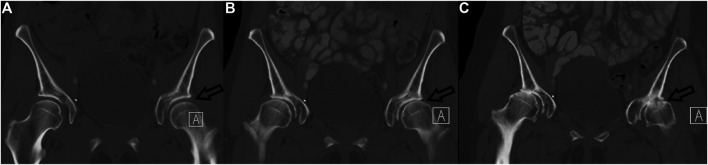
Radiation-related bilateral femoral head necrosis. Three coronal CT images of hip over 9 years follow-up are presented (2009–A, 2016–B, 2018–C). Note rapid bilateral hip osteoarthritis development, from normal (A) to bilateral femoral head necrosis, which is better seen on the left (C–arrow).
